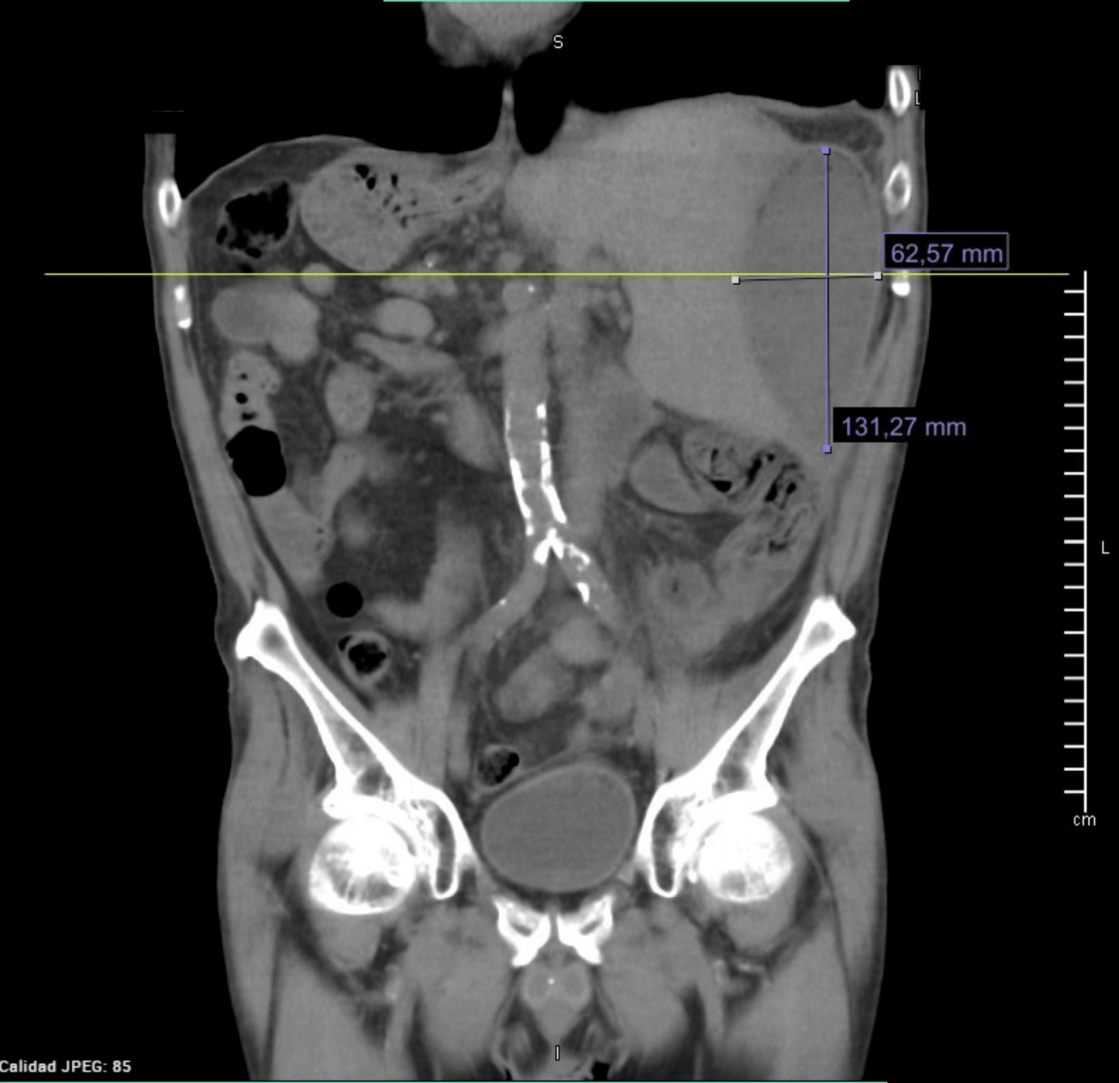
Coronal CT scan with IV contrast showing a hepatic abscess measuring 131.27 × 62.57 mm.
